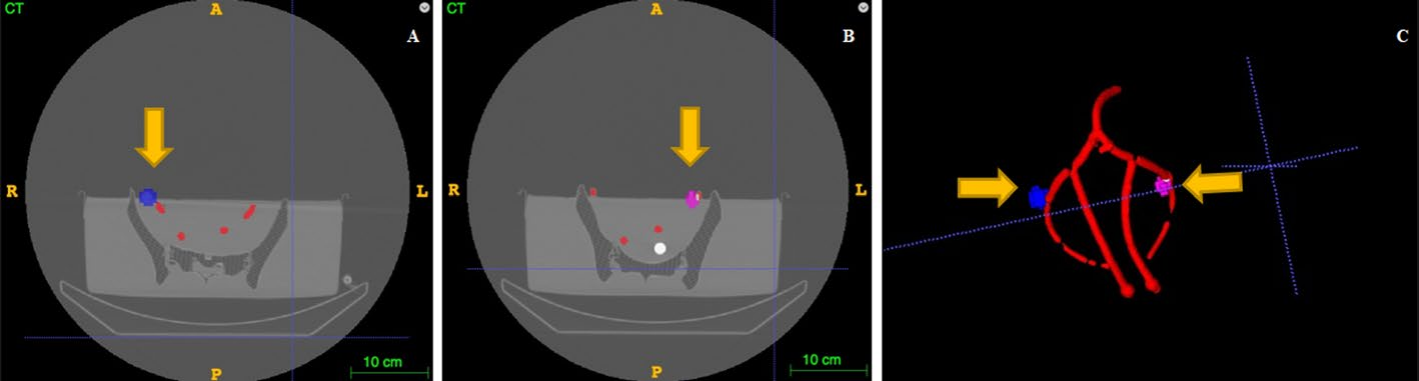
Registration of SPECT/CT and CT images using itk-SNAP® 3.6.0. The fusion CT image with the right (A) and left (B) SLN after loading of the SPECT/CT and after the synchronization of the CT with the SPECT/CT, achieved by the image fine-tuning over the pelvis. 3D reconstruction of vascular tree and both pelvic nodes in the same reference area (C). SPECT/CT, single-photon emission computed tomography combined with computed tomography; CT, computed tomography; SLN, sentinel lymph node
